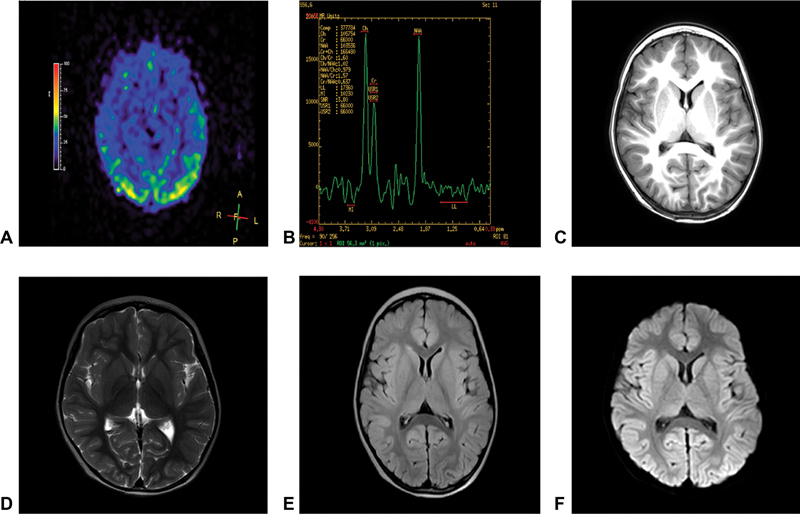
The magnetic resonance imaging of the proband. (
